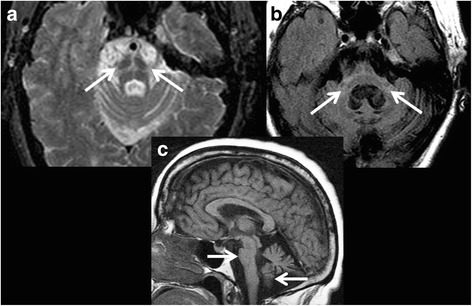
MRI of a patient with a clinical diagnosis of Multiple System Atrophy-*C*. a Axial proton density sequence at the level of the pons demonstrates cruciform pontine T2 hyperintensity consistent with the ‘hot cross bun’ sign secondary to selective vulnerability of the pontocerebellar tract in Multiple System Atrophy-*C*. Disproportionate atrophy of the pons and partially visualized cerebellar hemispheres is also evident. b Axial FLAIR sequence with cruciform T2 hyperintensity within the pons and middle cerebellar peduncles (i.e., ‘Middle Cerebellar Peduncle sign’) with marked atrophy. Cerebellar hemispheric and vermian atrophy is also seen with *ex vacuo* dilatation of the fourth ventricle. c Sagittal T1 sequence showing marked atrophy of the brainstem and cerebellar vermis
